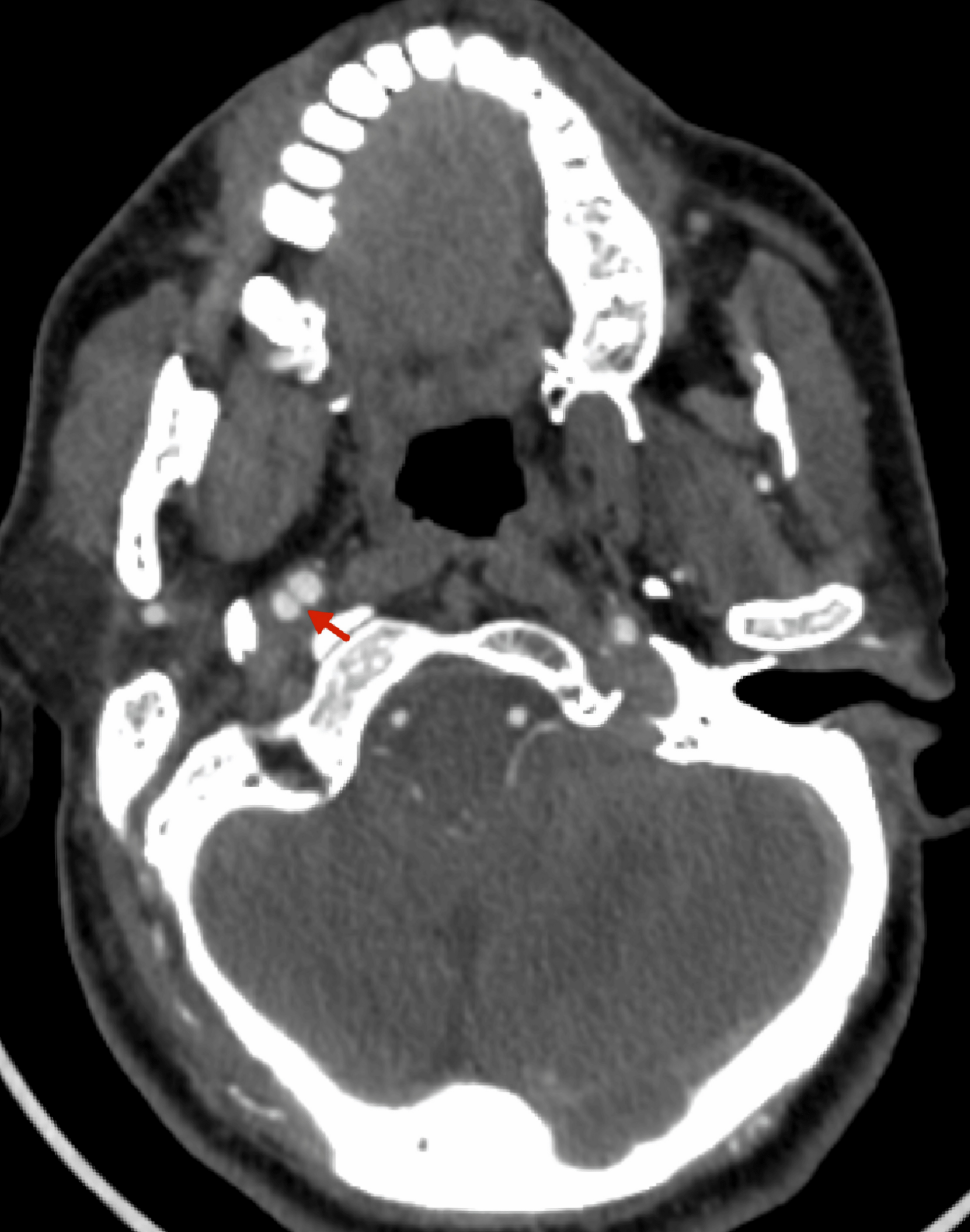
Computed tomography angiography (CTA) of the head and neck revealed an extracranial right internal carotid artery dissection, with the main feature being an intimal flap tear and a double lumen sign (red arrow), indicative of false lumen formation.
